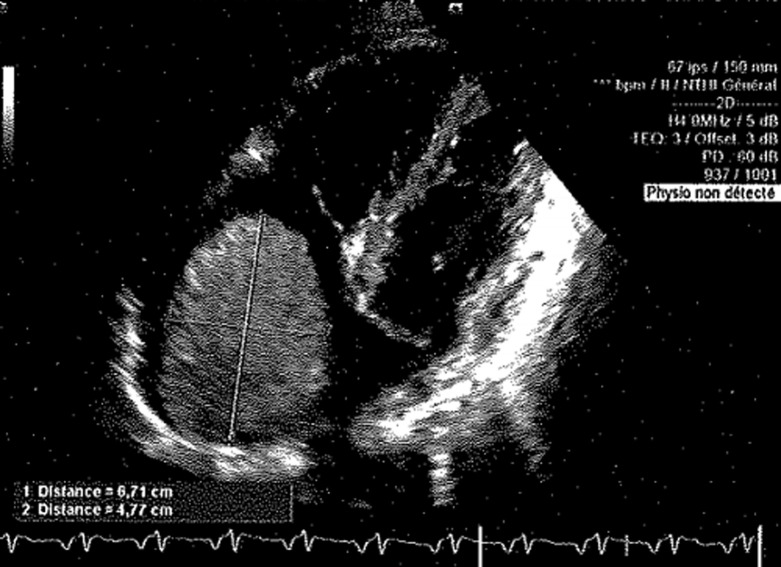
transthoracic echocardiography, other view showing the right atrial mass
